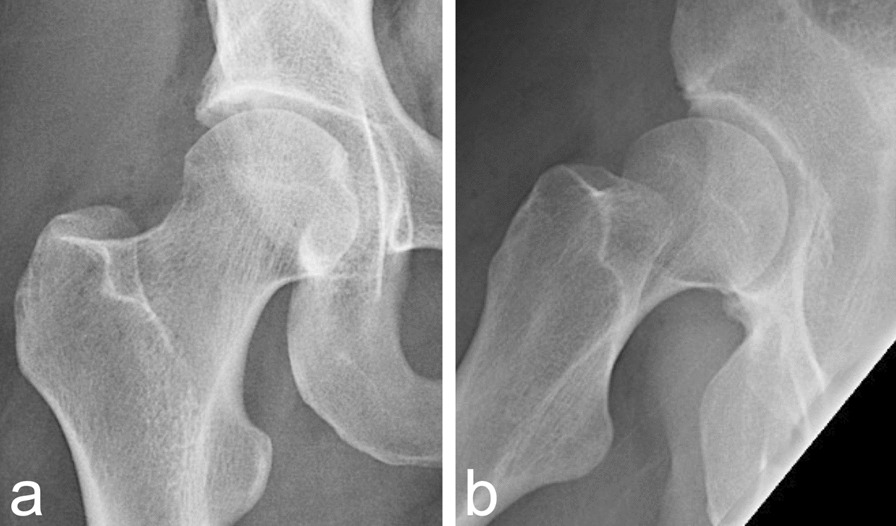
a, b Postoperative imaging of right hip (a, b). Pelvis anteroposterior radiograph and frog-lateral view with spherical femoroplasty
